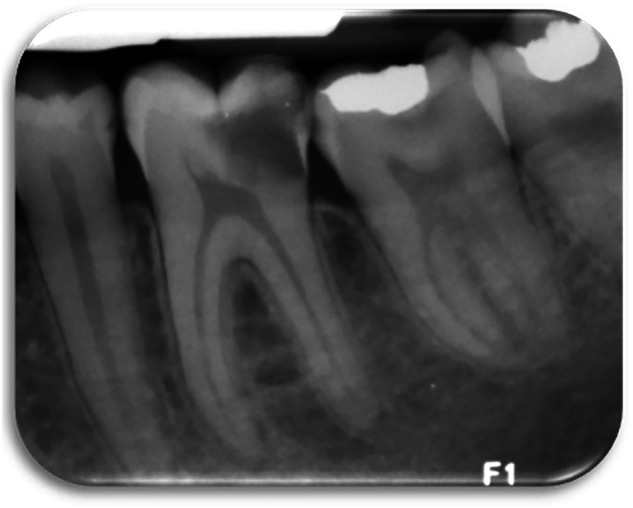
Periapical radiograph of lower left quadrant.
